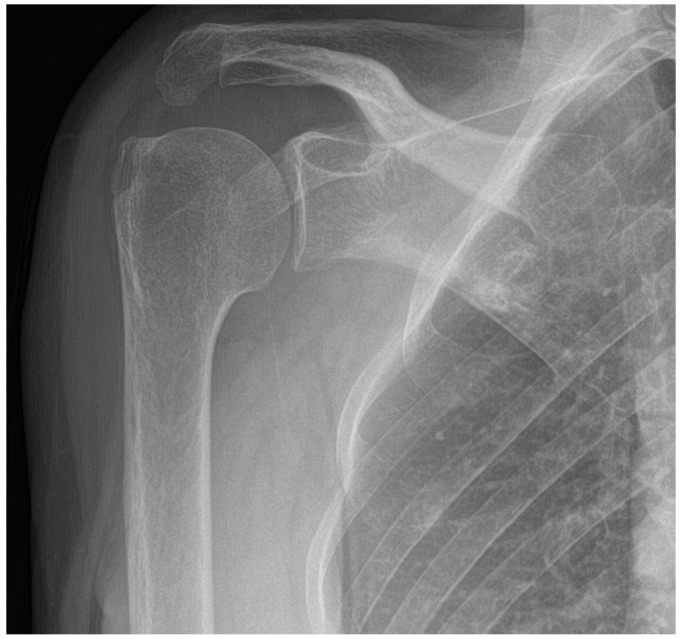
Radiograph of the right shoulder in extrarotation showing mild acromioclavicular arthrosis.
